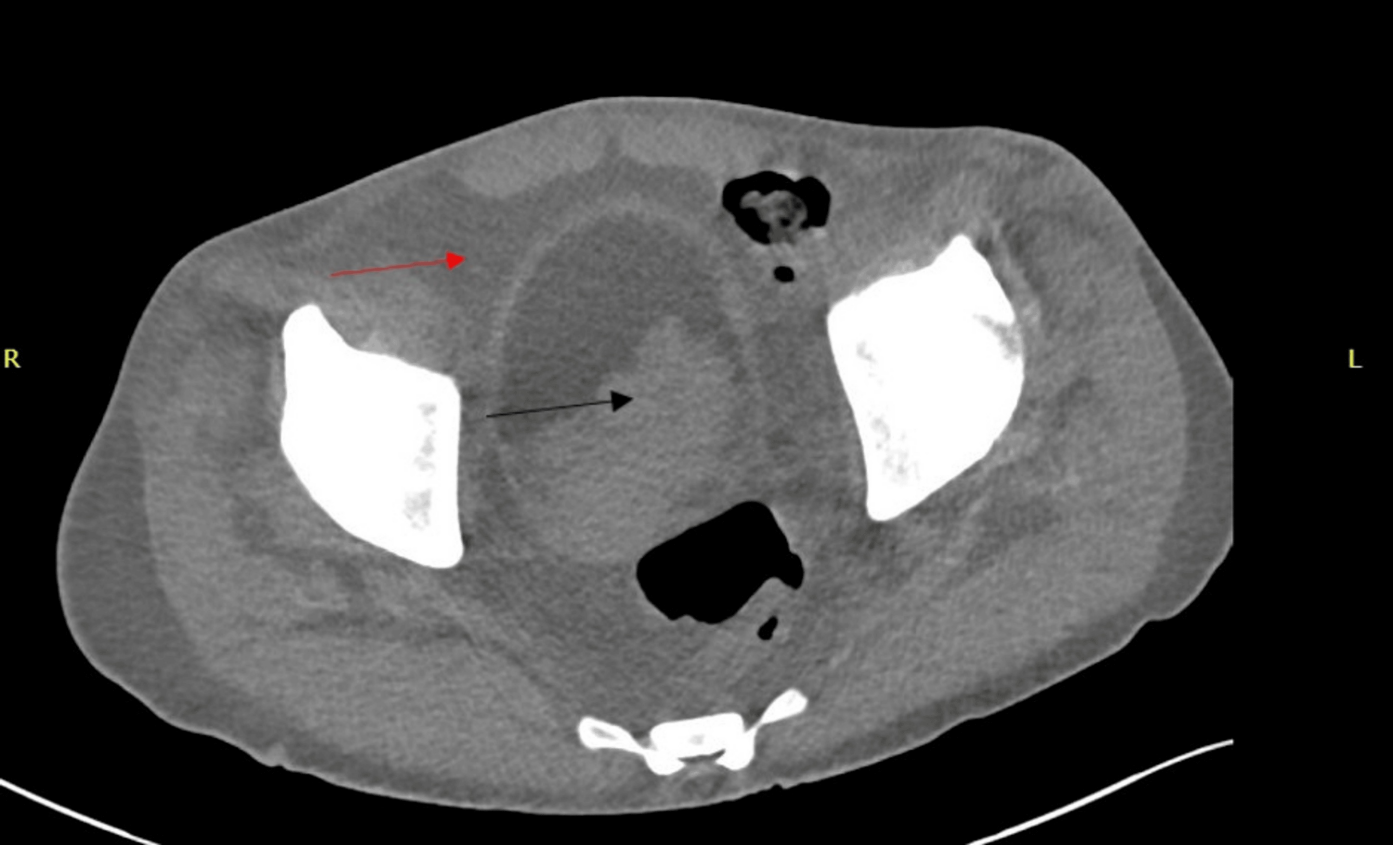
Non-contrast CT abdomen and pelvis Severe bilateral hydronephrosis and hydroureter to the level of the pelvic mass. Interval increase in size of the mass (black arrow) in the region of the prostate compatible with disease progression. The cystic component of the mass is poorly evaluated secondary to limitations of the study. Moderate ascites (red arrow). CT: computed tomography
